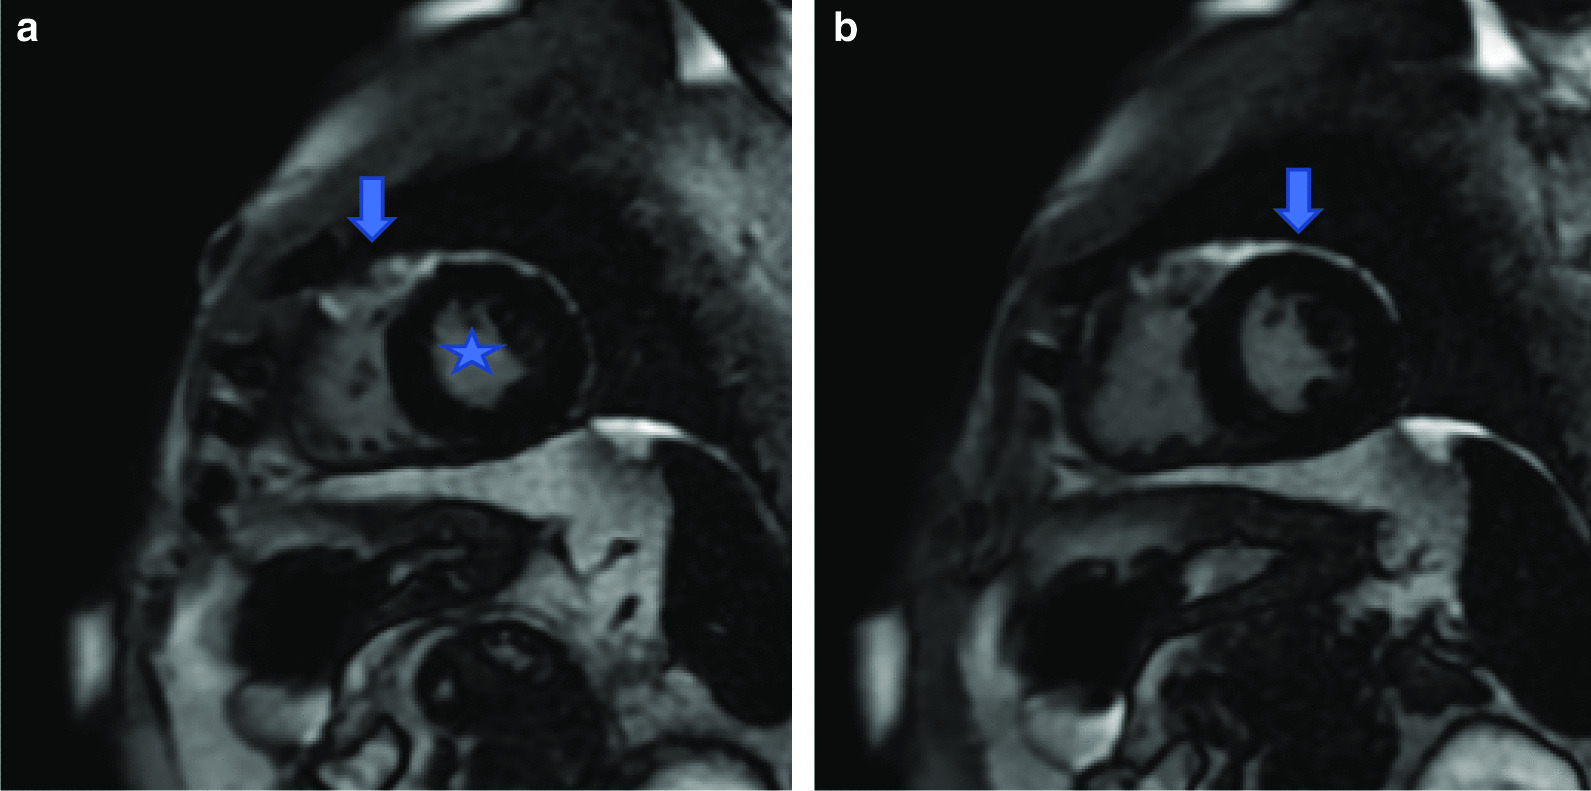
Cardiovascular magnetic resonance imaging, fast gradient echo, short-axis view of the mid to apical segment showing late gadolinium enhancement of the free wall of the right ventricle (a) and pericardium over the anterior wall, lateral wall (b) (arrows). Star denotes left ventricular cavity. Star denotes the left ventricle
